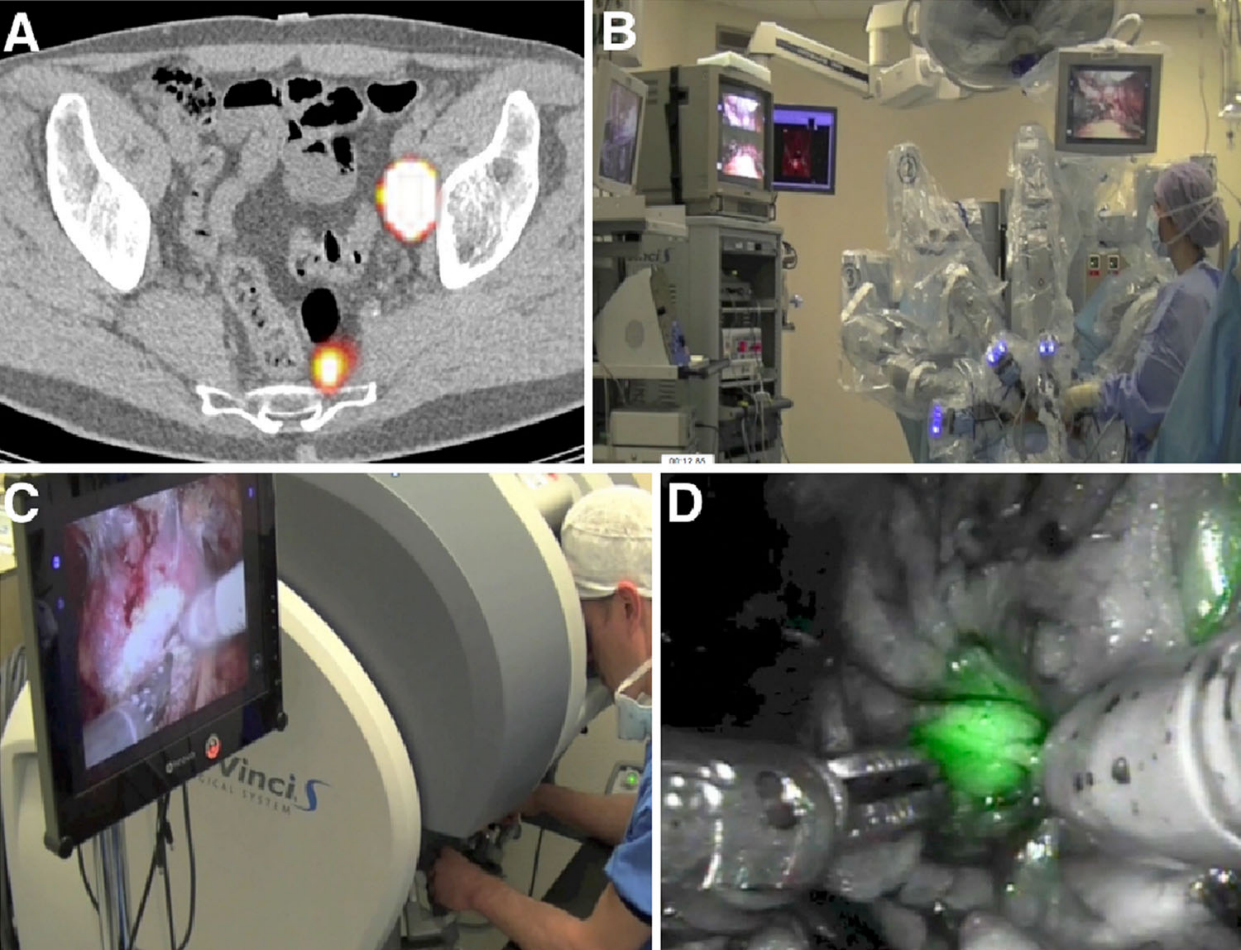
Following administration of the hybrid tracer ICG-^99m^Tc-nanocolloid in both lobes of the prostate, transversal SPECT/CT (a) shows iliac and presacral sentinel nodes, which are subsequently removed by means of a robot-assisted procedure (b–d)
